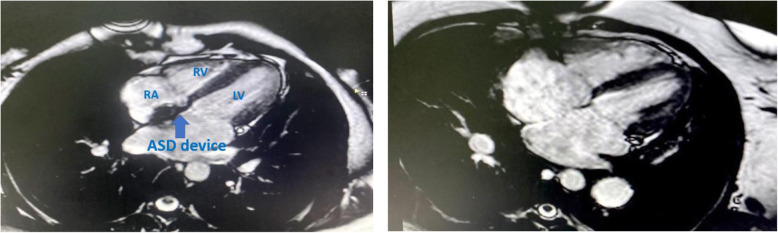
Cine SSFP 4 chamber MRI view in transcatheter closed case by ASO
